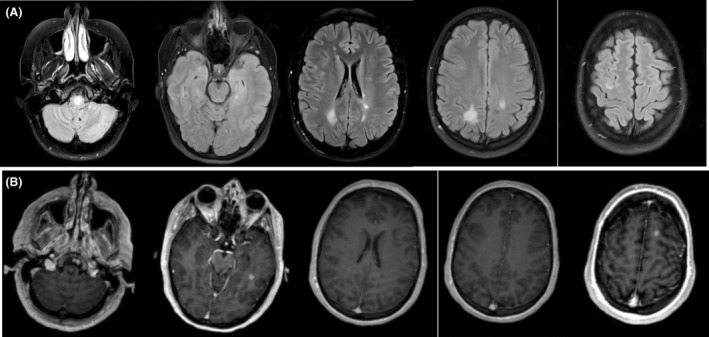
MRI brain with and without contrast on admission: (A) T2 FLAIR sequence showing hyperintensitiesy in the ventral medulla, central pons, and confluent periventricular and cortical hyperintensities. (B) T1 post‐contrast sequence showing enhancement of the medullary lesion, left inferior temporal lobe, and left frontal lobe.
